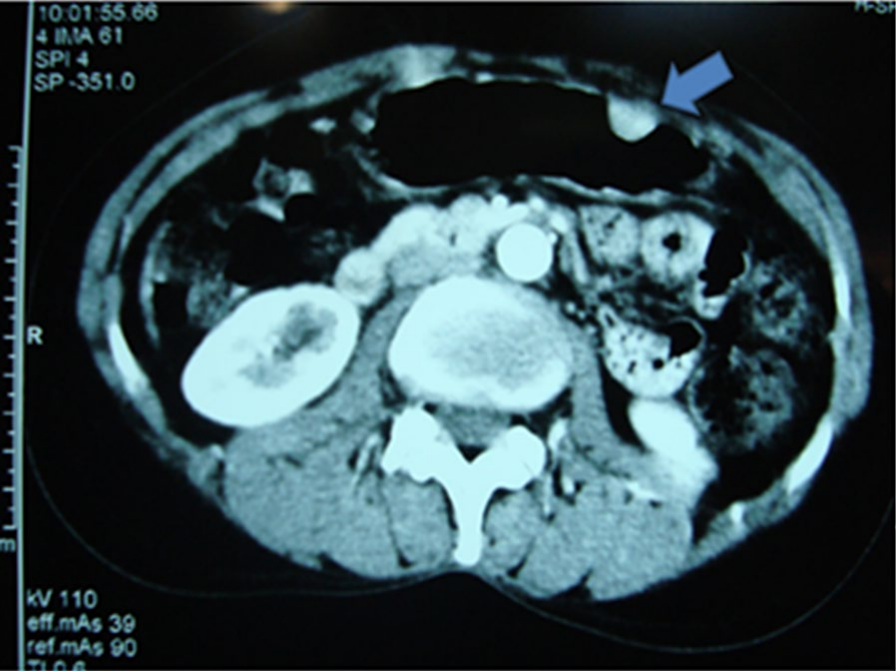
Contrast CT abdomen showing enhancing mass on the anterior gastric wall
